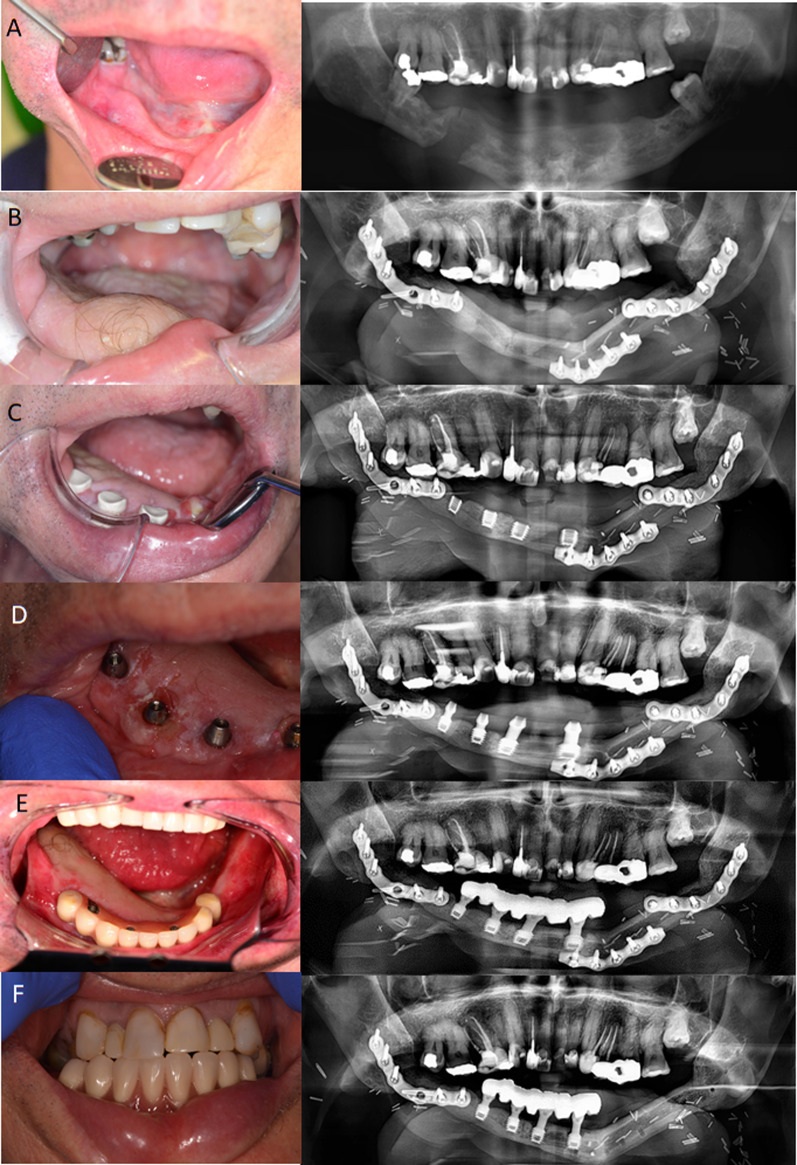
Clinical photos and panoramic radiographs of a 60 6-year-old male patient diagnosed with mandibular osteoradionecrosis with mandibular reconstruction. A Intraoral photo and panoramic radiography before reconstructive surgery; B intraoral photo and panoramic radiography after mandible reconstruction; C intraoral photo and panoramic radiography after dental implant placement; D the stability of inserted implants were well after 6 months and implant abutments were installed; E fitting restorations are stable in situ after superstructure and dentures installment; F a stable occlusal relationship was confirmed after 5 years follow-up
